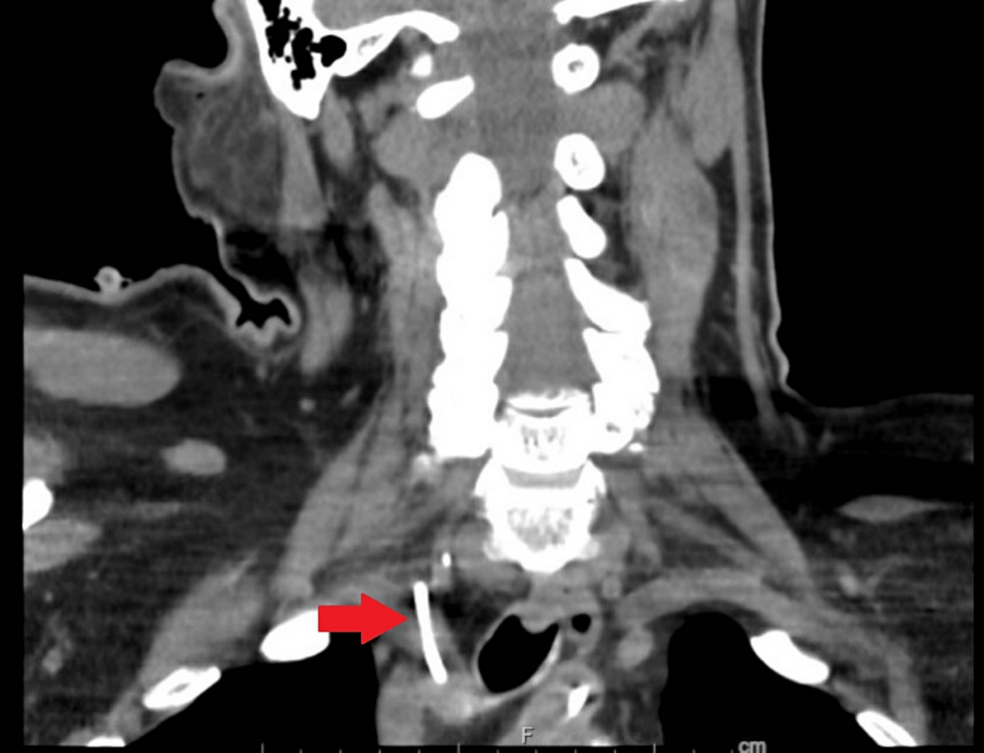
CT soft tissue neck demonstrating catheter traversing through the internal jugular vein to enter the subclavian artery
